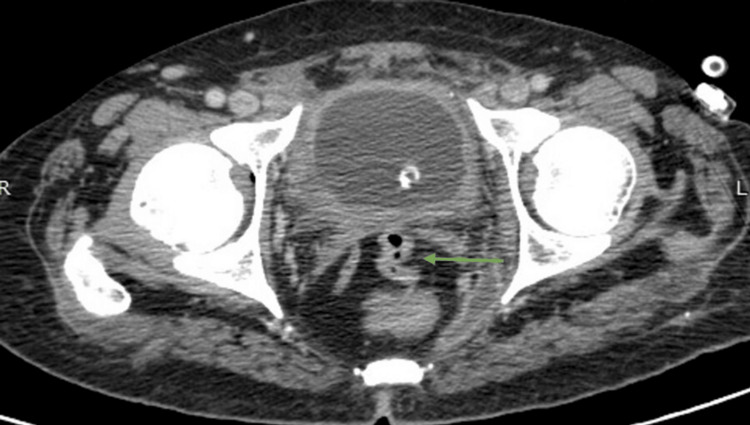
Contrast-enhanced CT KUB performed at eight weeks, demonstrating significant reduction in intramural gas and healing of the bladder wall, with a small residual anterosuperior bladder wall dehiscence (arrow). CT KUB: Computed tomography of the kidney, ureter, and bladder.
